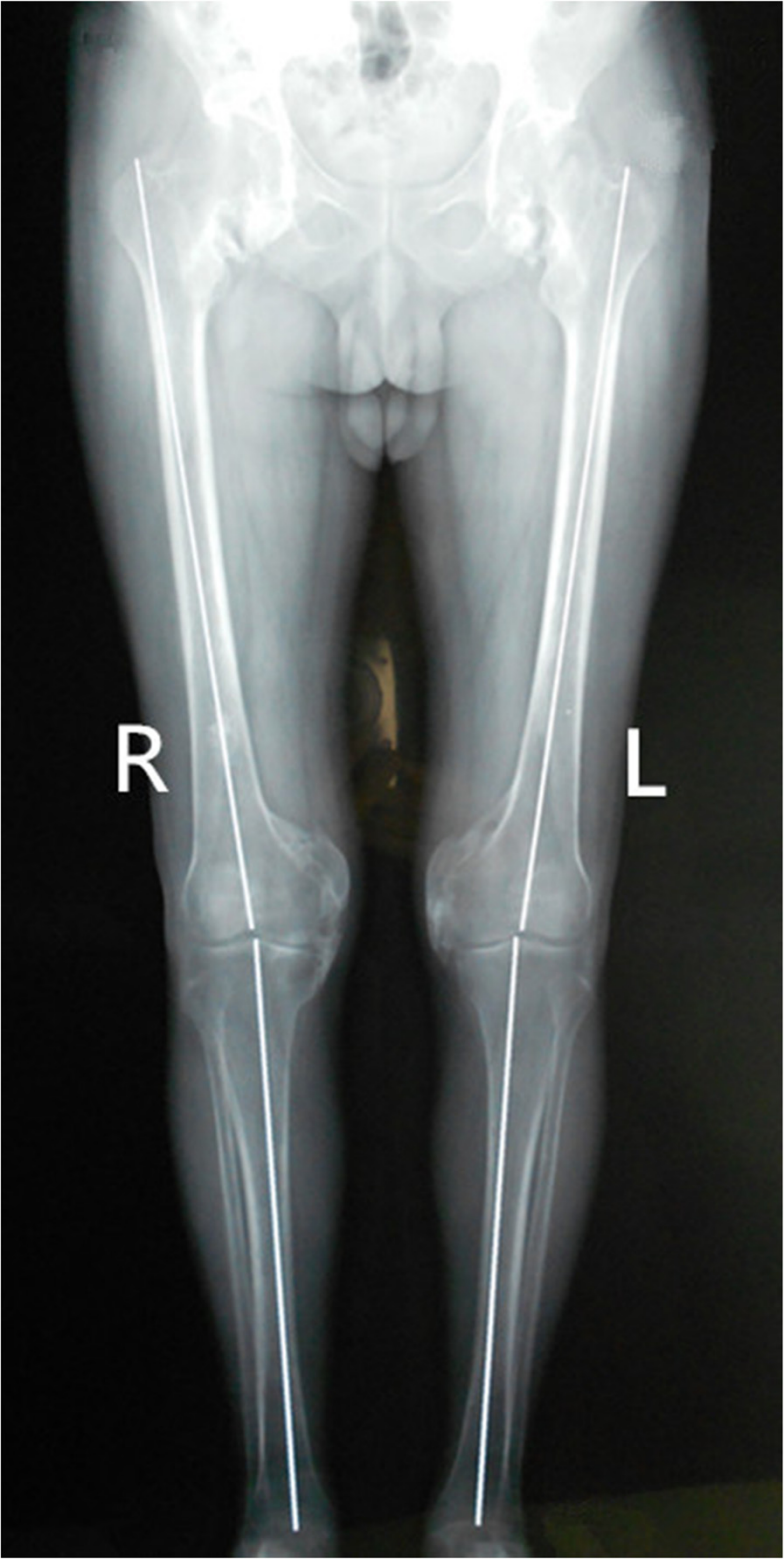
Preoperative radiographs of both lower extremities shows Lesions of four joints.
